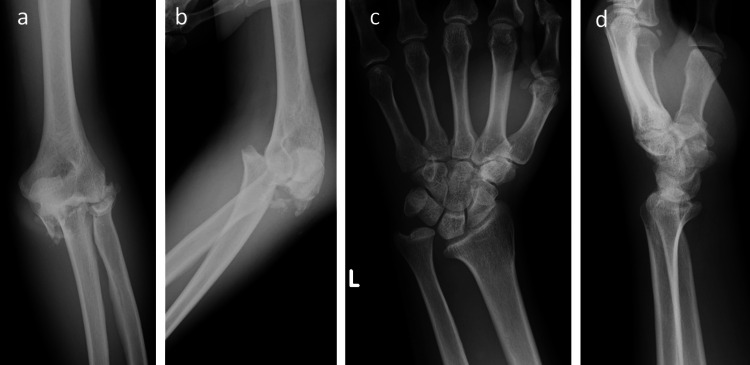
Preoperative X-ray images. (a) Anteroposterior and (b) lateral plain radiographs of the left elbow at initial presentation. These figures show a fracture-dislocation of the elbow. (c) Anteroposterior and (d) lateral radiographs of the left wrist. These figures show dislocation of the distal radioulnar joint.
